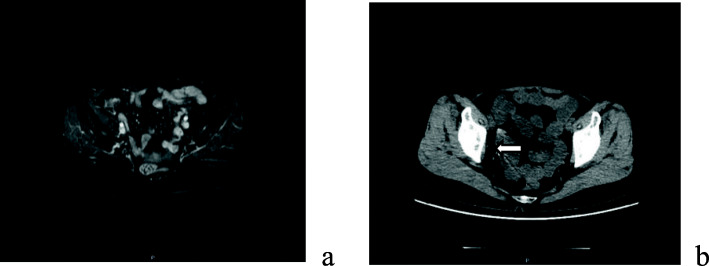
Postoperative image in June 2019: a. MRI showed no obvious local lesion. b. CT showed tumor disappeared, with only radioactive seeds remaining
